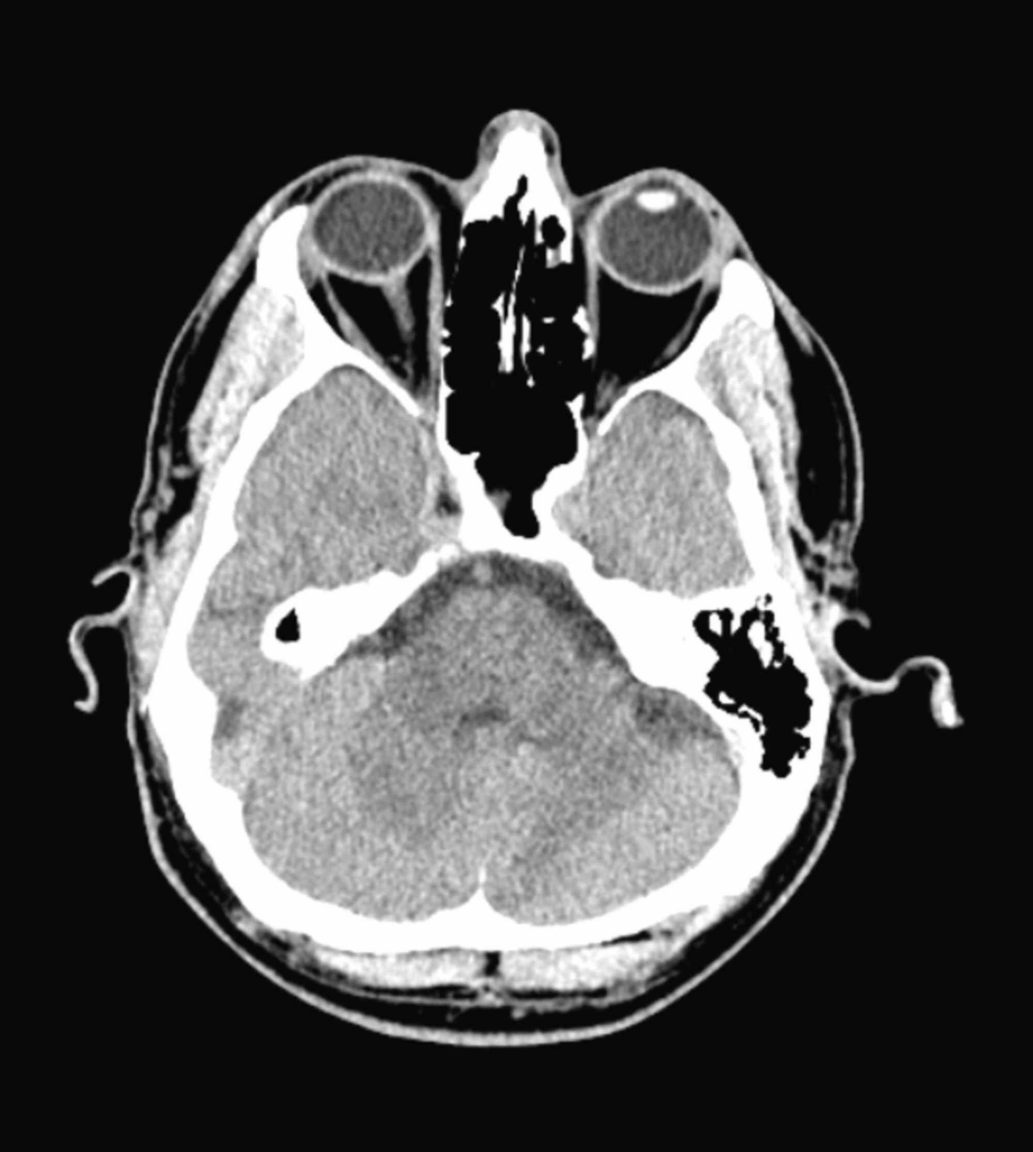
Computed tomography of the head
